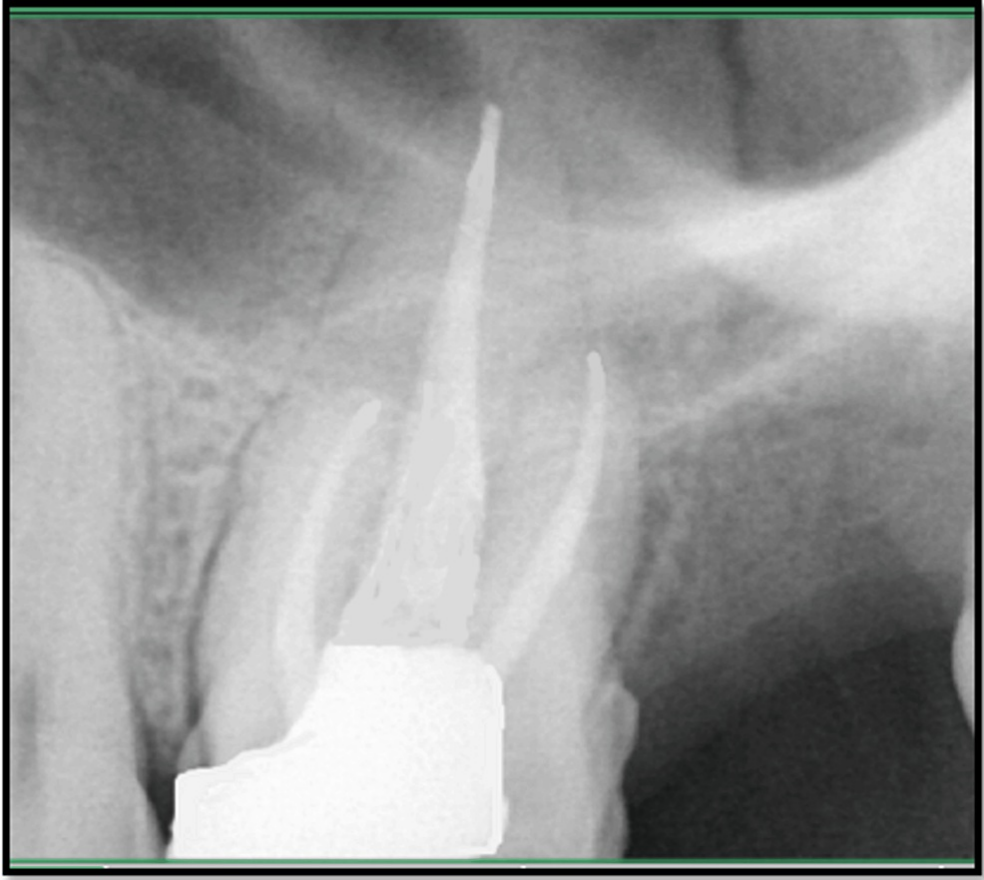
One-year follow-up radiograph showing sufficient healing.
